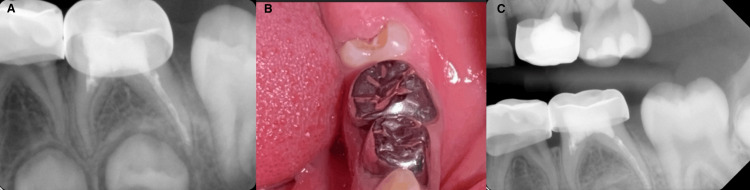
Postoperative clinical PA and BW radiographs after nine months showing complete resorption of Vitapex, without signs, symptoms, evidence of infection, lesion, or mobility A: Bitewing radiograph nine months postoperative for follow-up; B: Clinical intraoral photo nine months postoperative for follow-up; C: Periapical radiograph nine months postoperative for follow-up
